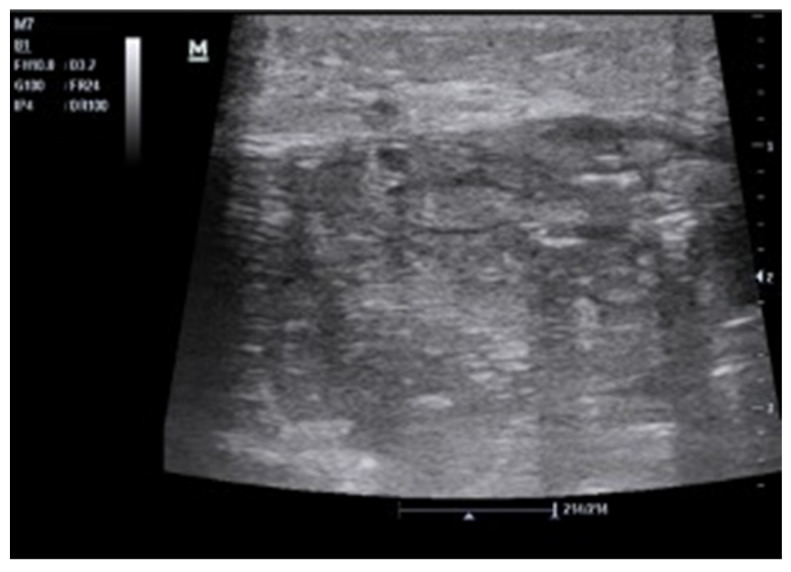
Black where it should not be. A 35-year-old man with a significant history of intravenous drug abuse presented with fever and local pain in the right forearm. The arm was warm and with tight skin. The ultrasound showed a necrotizing fasciitis with subcutaneous thickening, air, and fascial fluid. He underwent decompressive fasciotomy in association with large spectrum antibiotic therapy.
